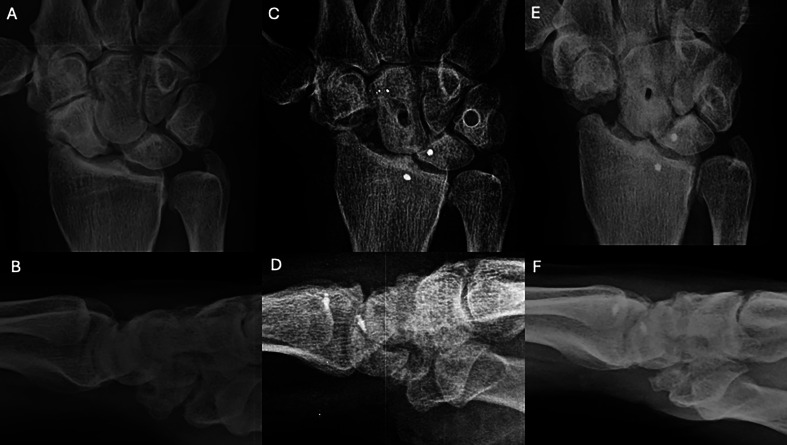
Clinical case of ECRB Transosseous C-T and Scaphoid excision. A and B. Preoperative wrist X-rays of SLAC stage II (AP and Lateral views). C and D. Postoperative wrist X-rays (AP and Lateral views) after 2 months. E and F. Postoperative wrist X-rays (AP and Lateral views) after 2 years. Source: Dr. Emmanouil Apergis.
